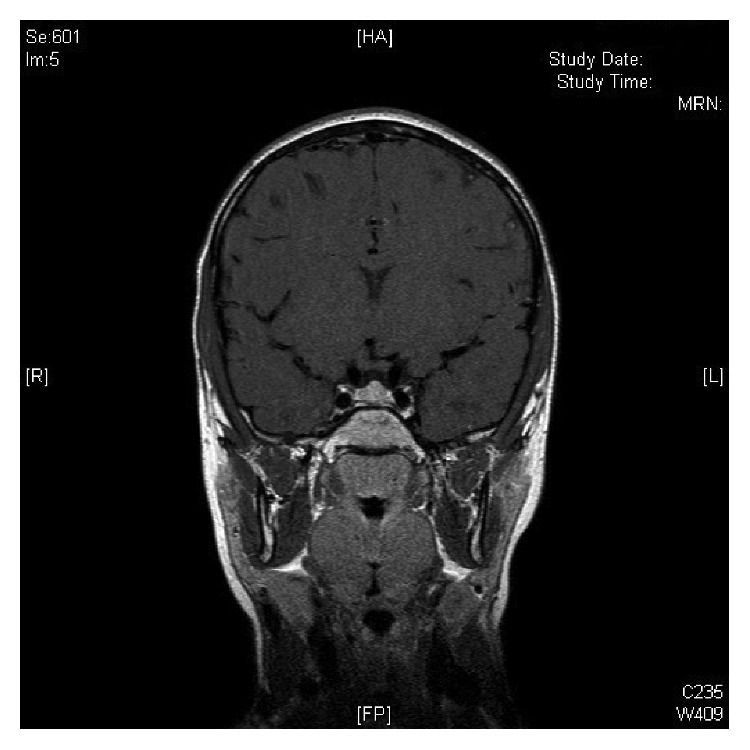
Coronal post-Gadolinium brain MRI image showing a normal pituitary morphology, of pubertal size, with marked upper surface convexity.
